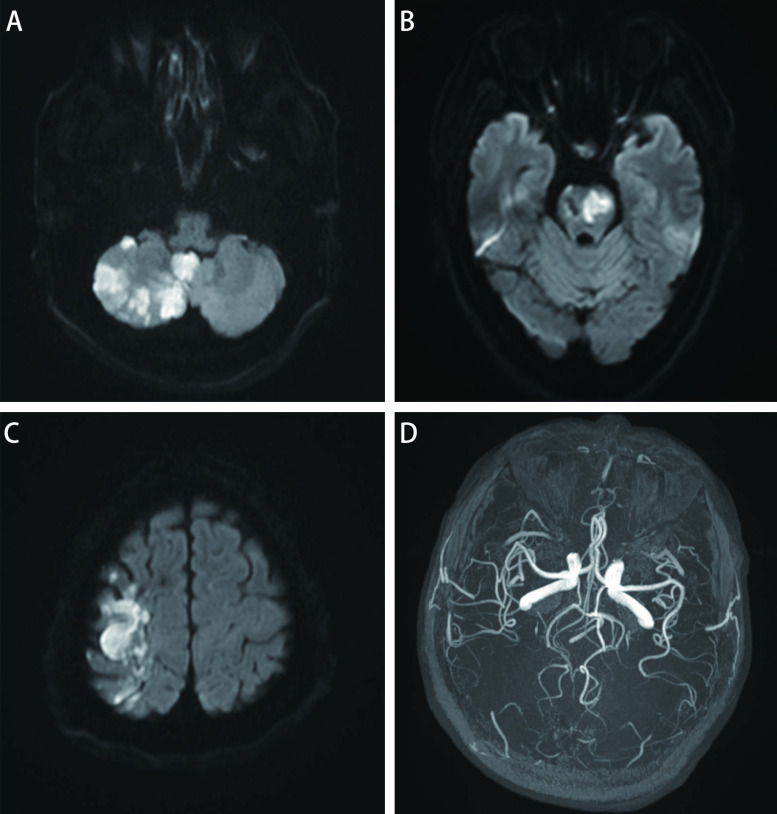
病例19的DWI及MRA图像特征。A-C：右侧额叶、顶叶、脑桥、右侧小脑半球及扁桃体可见多发高信号，提示梗死；D：颅内动脉管腔未见明显狭窄 DWI and MRA feature of case 19. A-C: DWI shows multiple foci of high signal intensity in right frontal lobe, parietal lobe, pons, right cerebellar hemisphere and tonsil, indicating infarction; D: MRA shows no significant narrowing of all the intracranial arterial lumen.
